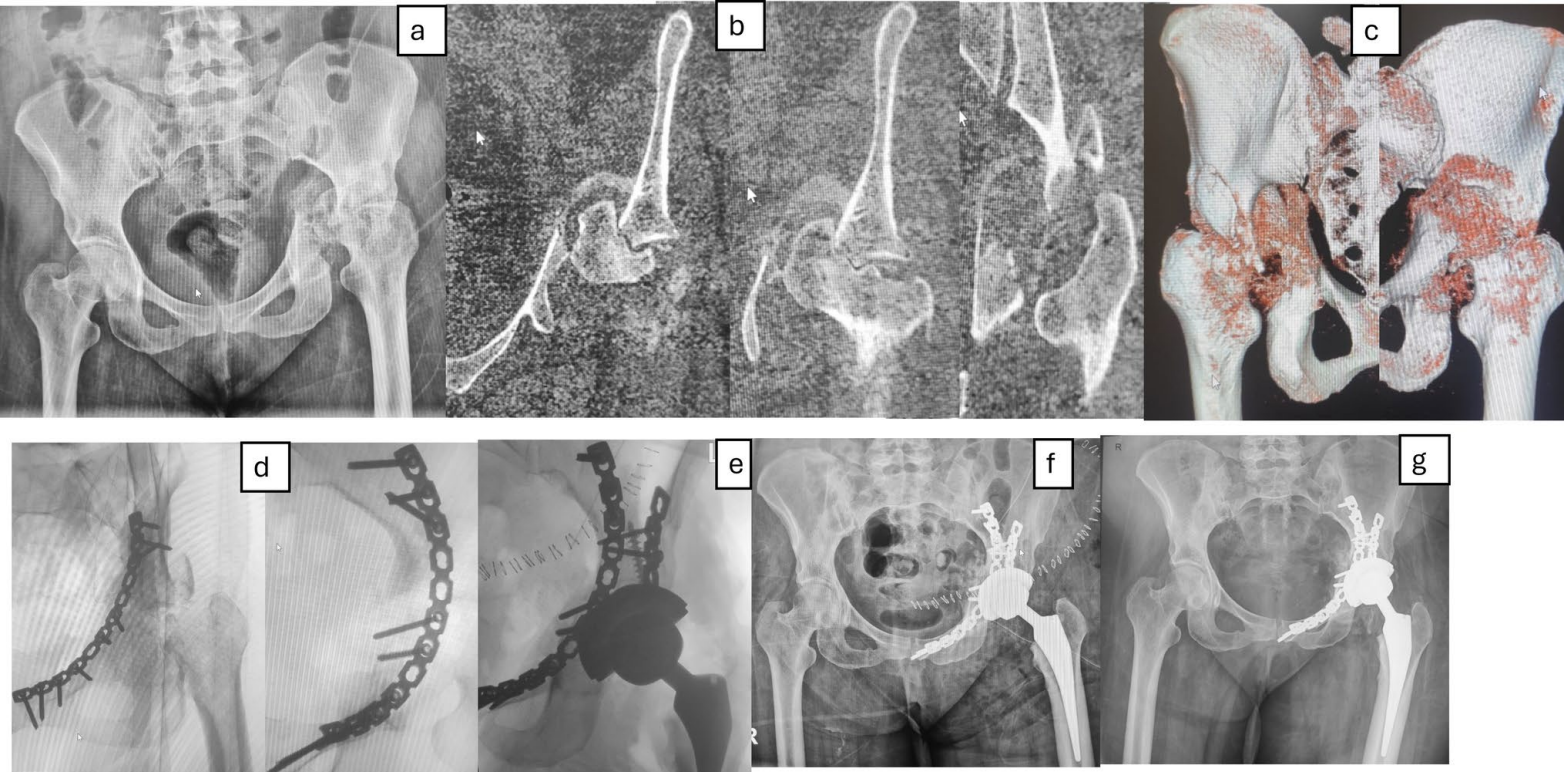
a–c Preoperative anteroposterior radiograph, axial CT cuts, and 3D CT reconstruction demonstrating a 7-week neglected transverse posterior wall fracture with central hip dislocation and an indentation fracture of the femoral head at the fracture edge. Intraoperative oblique fluoroscopic views during the ilioinguinal approach, showing removal of surrounding callus, intrapelvic femoral head osteotomy (head irreducible), fracture mobilization, fixation of the anterior column, buttressing of the quadrilateral plate, fixation of the posterior wall, and application of a primary cementless hip replacement. f, g Immediate postoperative and final follow-up radiographs demonstrating a stable acetabular cup without migration or radiolucent lines
